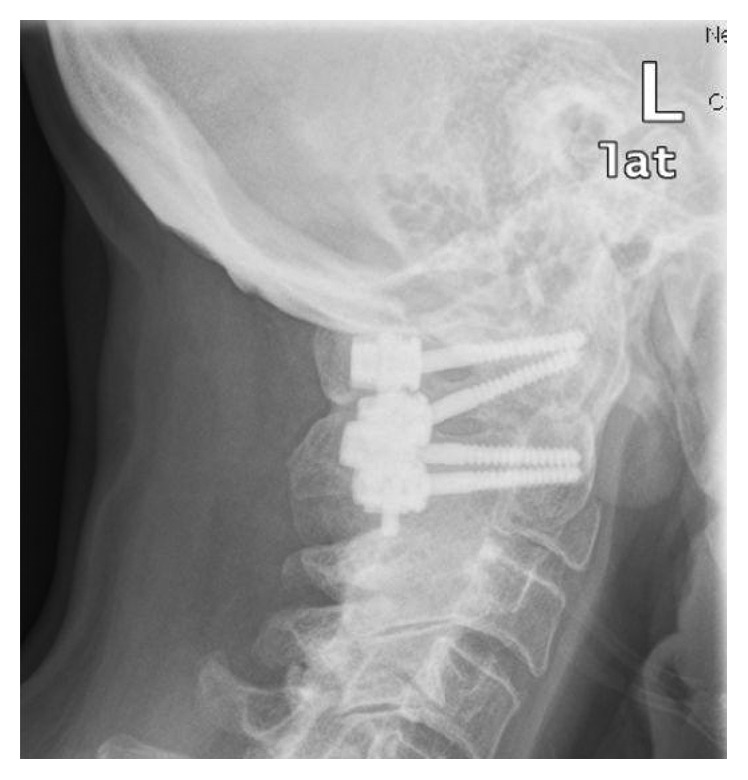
Lateral X-ray of the neck after dorsal stabilisation. The inserted devices are in correct position in all plains.
